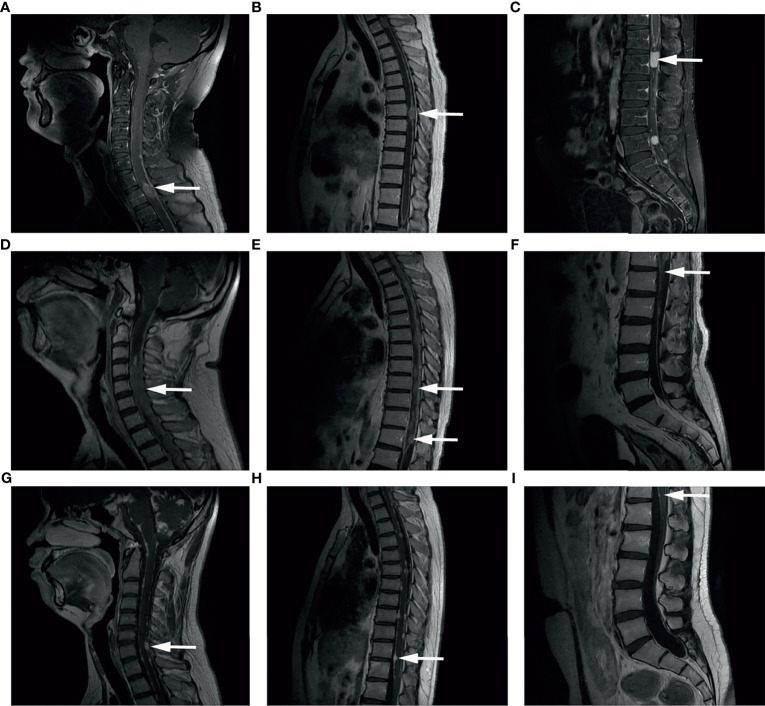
Representative images of Gd-enhanced MRI. Spinal dissemination presented as a hyperintense signal showing as mass or line (arrows) in the spinal canal in T1-weighted MR images. (A–C) Spinal Gd-MRI revealed multiple contrast-enhanced mass at the spine in a male aged 34 years old at MSD, who was initially diagnosed as GBM at the left occipital lobe. (D–F) Spinal Gd-MRI demonstrated thick linear and clumpy enhanced lesions along the whole spine in a male aged 40 years old at MSD, who was initially diagnosed as anaplastic oligodendroglioma (WHO grade III) at the left frontal lobe. (G–I) Spinal Gd-MRI demonstrated small enhanced lesion at the spine in a male aged 17 years old at MSD, who was initially diagnosed as astrocytoma (WHO grade II) at both cerebral hemispheres, cerebellar hemispheres, brainstem surface, ventricles, cisterns, and meninges.
